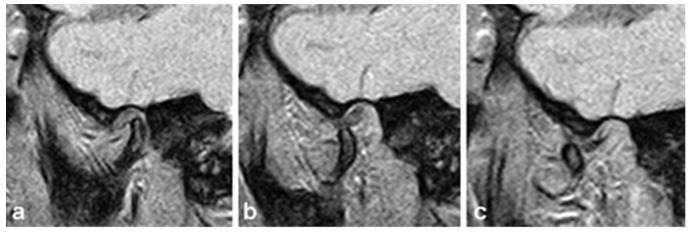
Sagittal view of TMJ on MRI. (a) Closed mouth position. (b) Semi-opened mouth position, 25 mm distance between upper and lower incisors. (c) Maximum mouth opening position.
